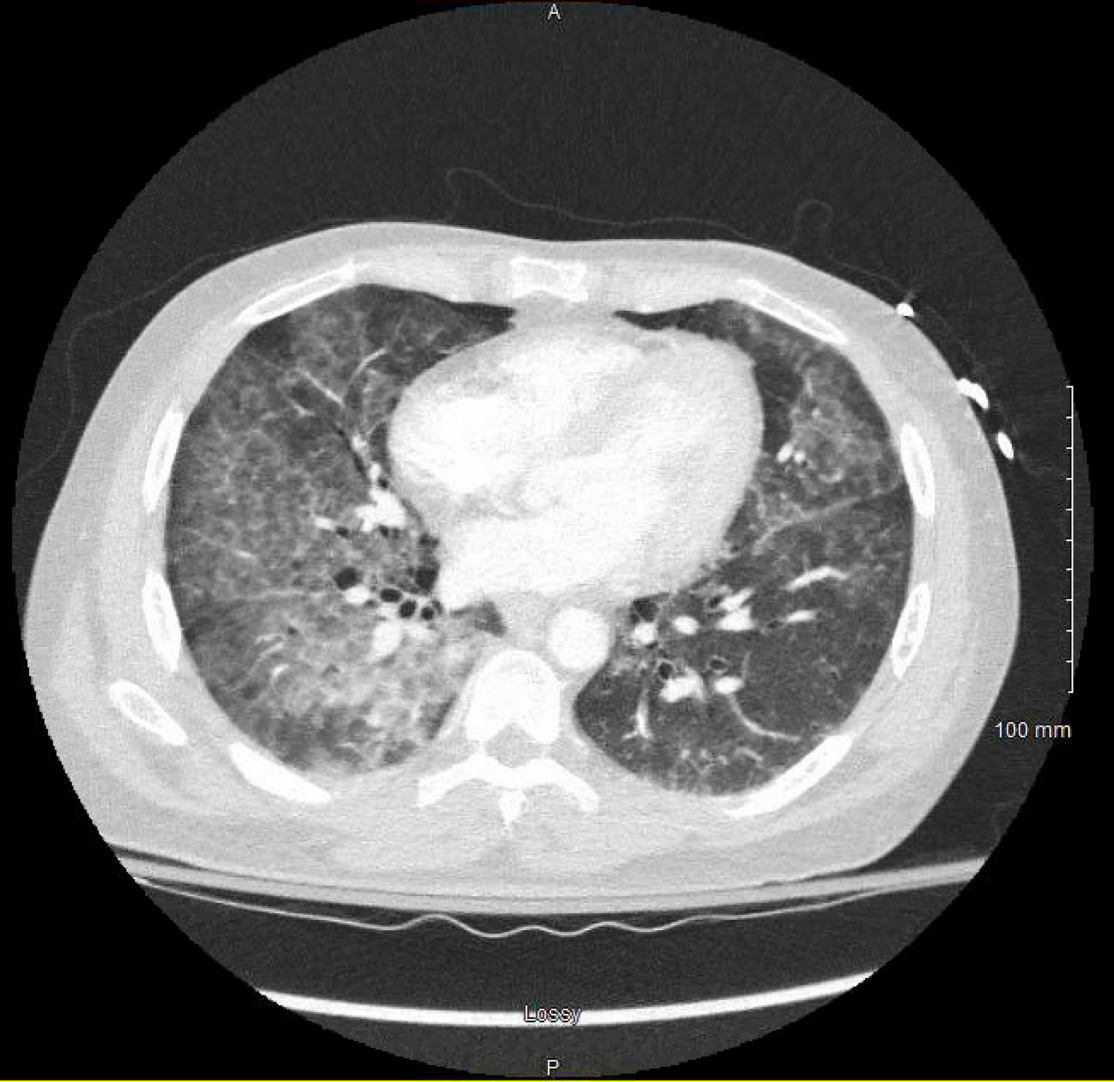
Chest CT showing ground-glass opacities
